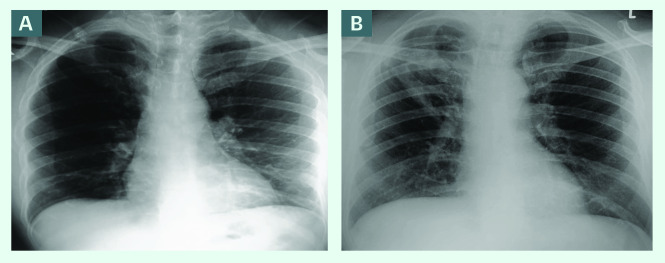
A) Chest X-ray from 2014 with normal findings, and B) from presentation in December 2020, showing fibrosis with cavitation in the upper zone on the right
